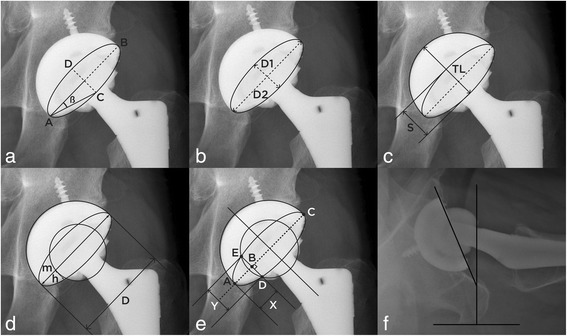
a Depiction of the method of Liaw et al. The *β* angle is used to calculate the anteversion. b Depiction of the method of Lewinnek et al. The long and short axes of the ellipse are used to calculate the anteversion. c Depiction of Widmer’s method. The short axis of the ellipse and total length of the acetabular component are used to calculate the anteversion. d Depiction of the method of Hassan et al. The long axis of ellipse and the head edge are used to calculate anteversion. e Depiction of the method of Ackland et al. Each perpendicular distance from the end of the ellipse to the cross section between the tangent and the diametrical line is used to calculate the anteversion. f Depiction of the method of Woo and Morrey. The cross-table lateral radiograph is used to calculated the anteversion
